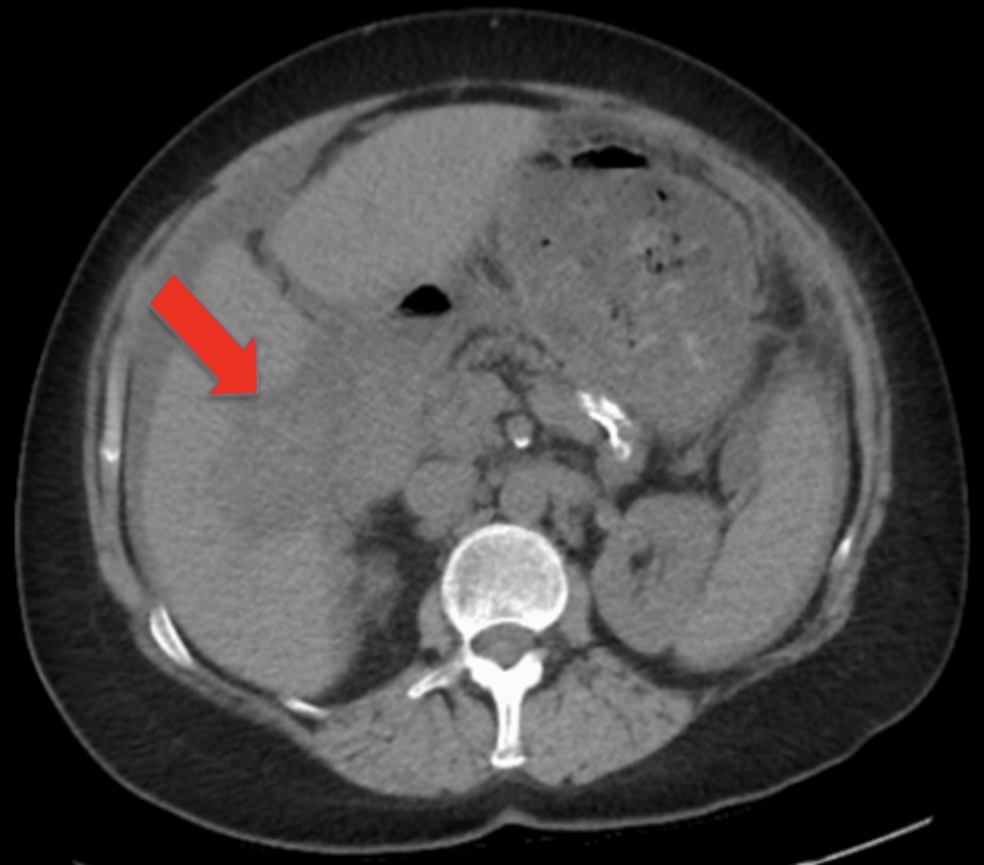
Initial CT scan of the abdomen and pelvis. The image demonstrates an ill-defined hepatic mass measuring approximately 5.9 x 3.9 cm with increased soft tissue attenuation noted along the posteromedial aspect of the surface of the right lobe of the liver.
